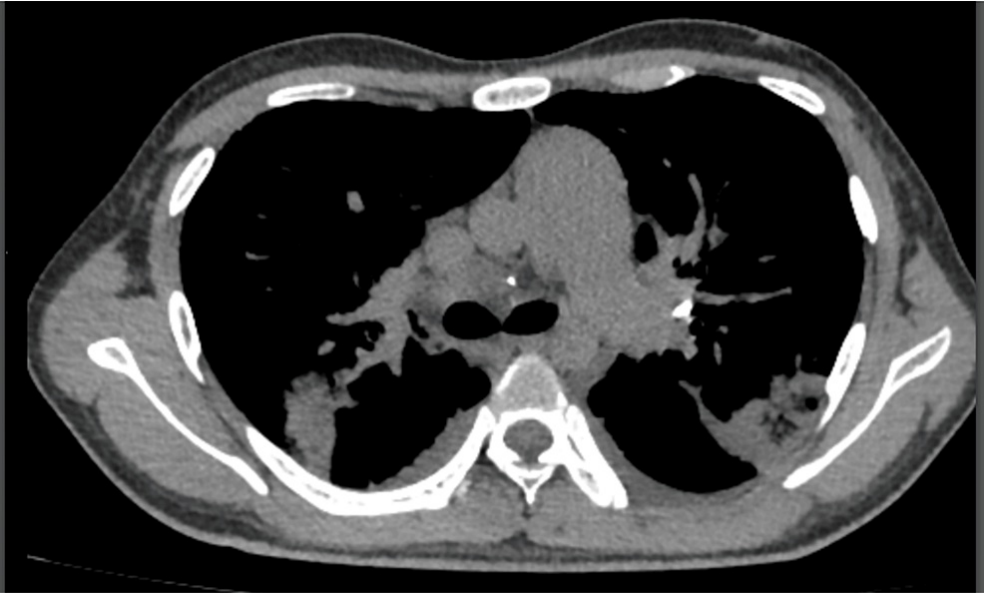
Chest computed tomography scan Posterior pulmonary atelectasis in both lungs and inferior lobes with consolidation zones
